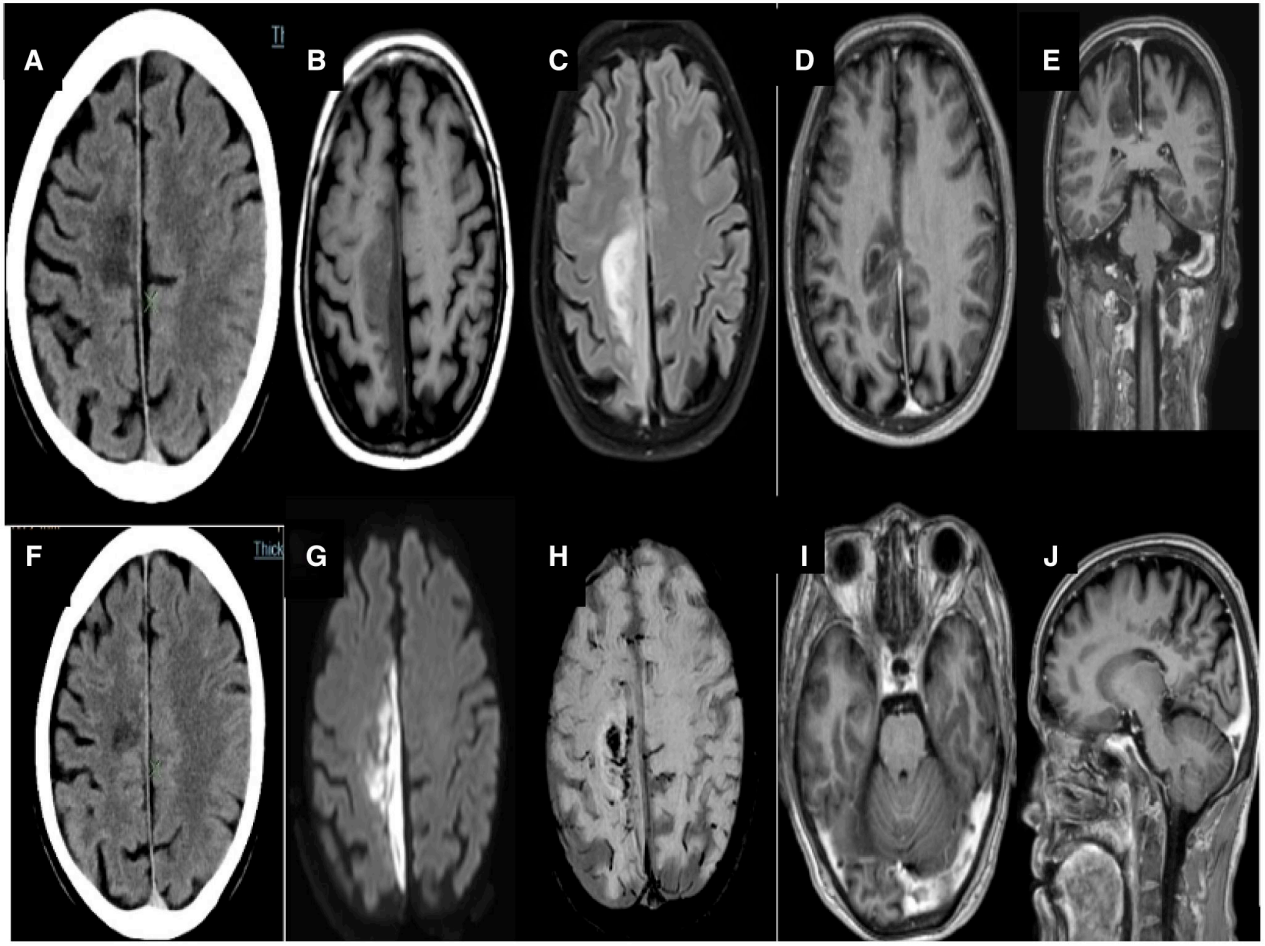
Axial CT showed ill-defined hypodense areas in the right para-falcine region without haemorrhagic component (A, F). Magnetic resonance images of the brain (B). T1-weighted axial image shows low signal intensity involving the right para-falcine region with corresponding high signal in T2-weighted axial image (C). Post contrast study shows minimal peripheral enhancement (D). Diffusion-weighted image (G) show high signal intensity involving the right parasagittal region with gyral haemorrhage (H). Post-gadolinium T1- weighted image displays a filling defect in the left sigmoid sinus (E, I, J).
